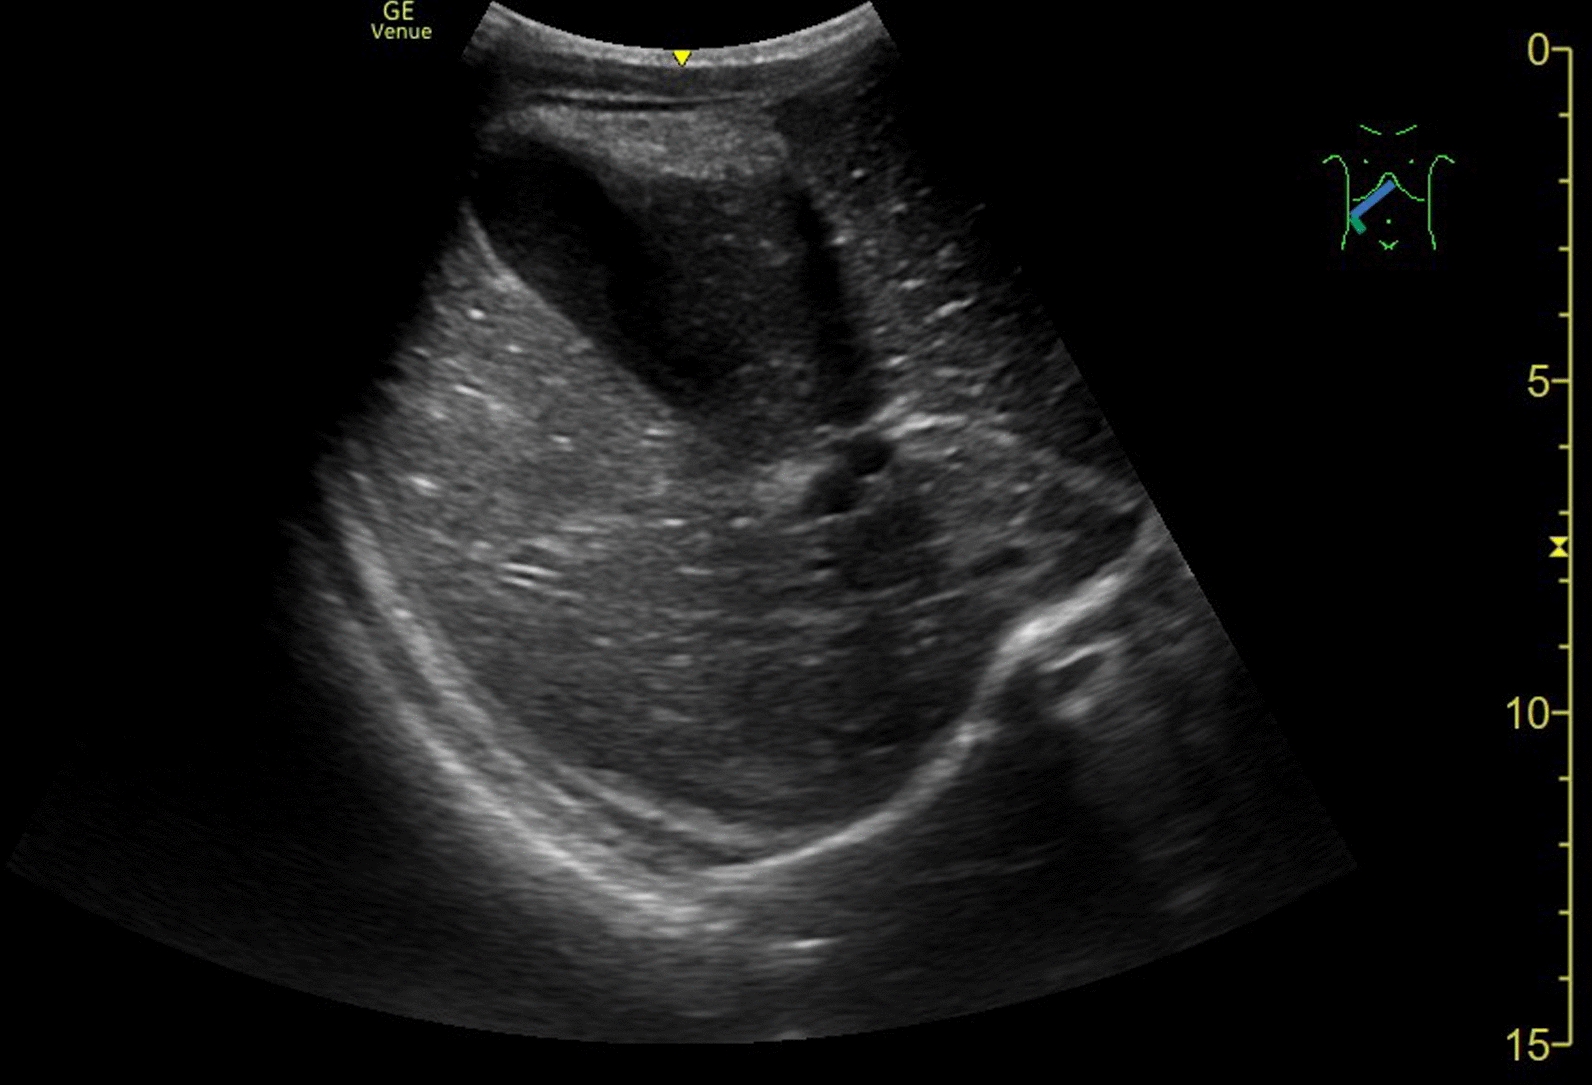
Overview of the liver and gallbladder as seen on transabdominal ultrasound
